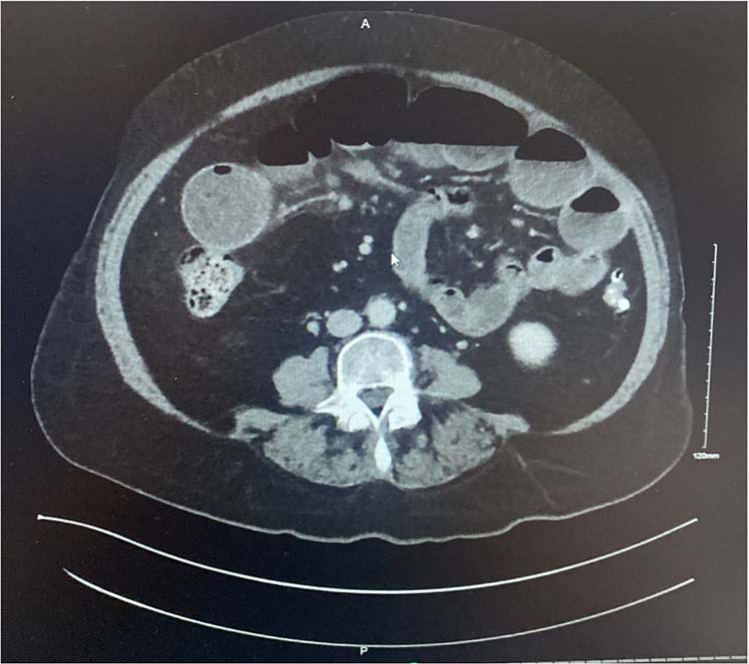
Axial section of contrast enhanced CT AP demonstrating intussusception with surrounding lymphadenopathy.
